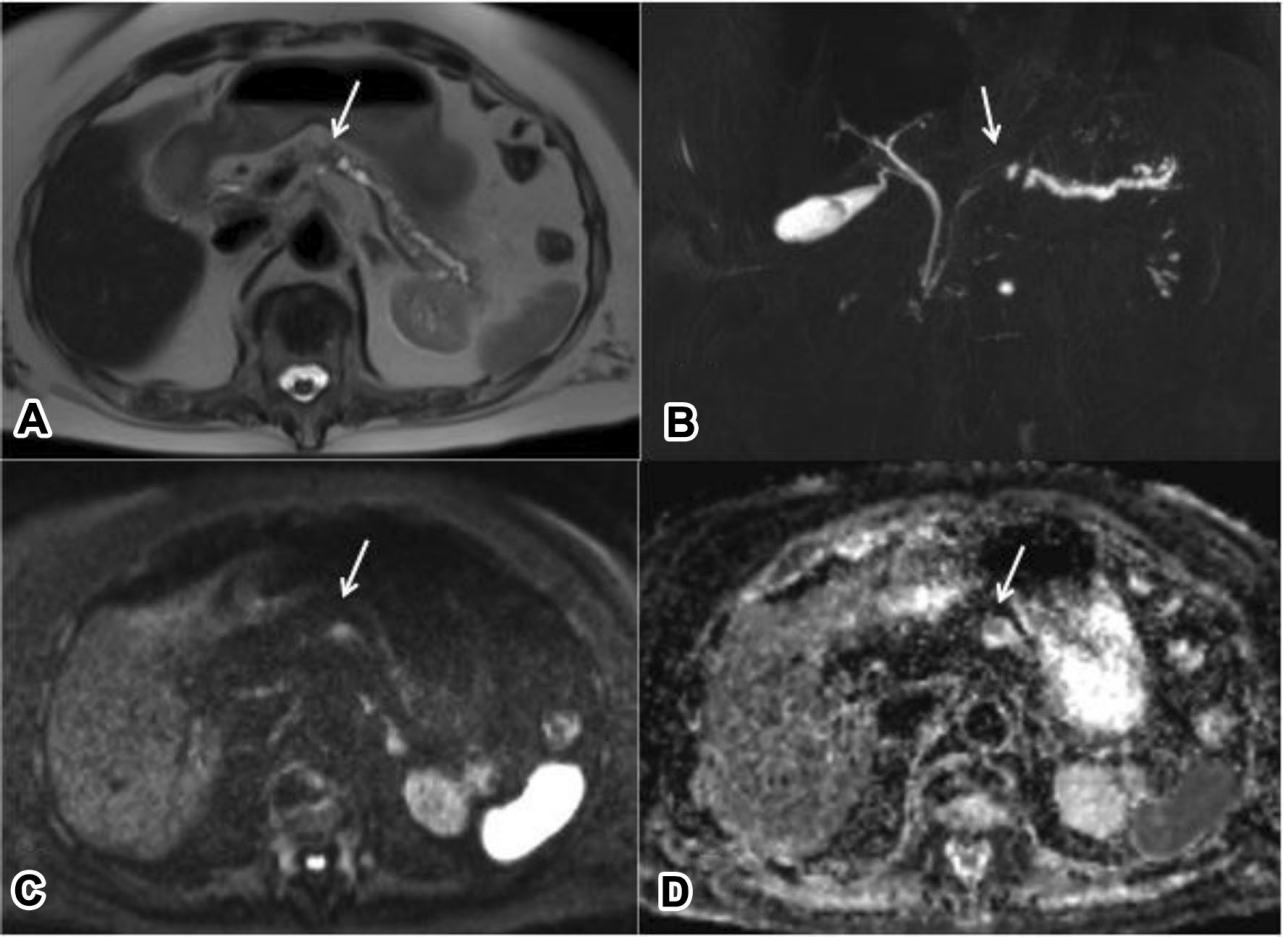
Small PDAC. At T2 HASTE (a) a moderate dilatation of mpd can be appreciated, with a stenosis at the body level (arrow). At MRCP (b) the stenosis of mpd (arrow) and its dilatation upstream is confirmed. At DWI (b 800) a small hyperintence spot is appreciable at the level of stenosis (arrow in C), which appears hypointense on ADC map (arrow in D)
